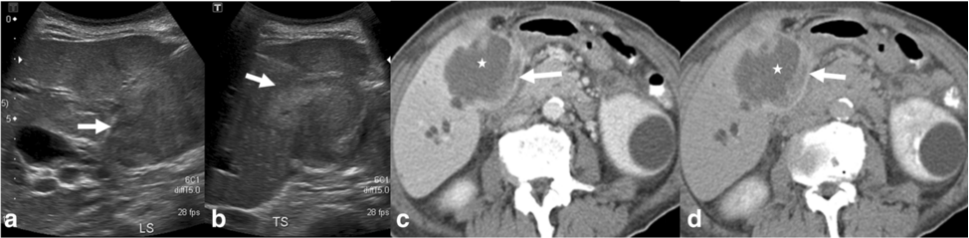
Gall bladder carcinoma. The gallbladder and gall bladder fossa is replaced by a large heterogeneous mass of mixed echogenicity on ultrasound (a, b) and heterogeneous hypodense mass (*) on contrast enhanced CT with enhancement of the fibrous stromal component (arrow) of gallbladder carcinoma during the portal venous (c) and delayed phases (d).
